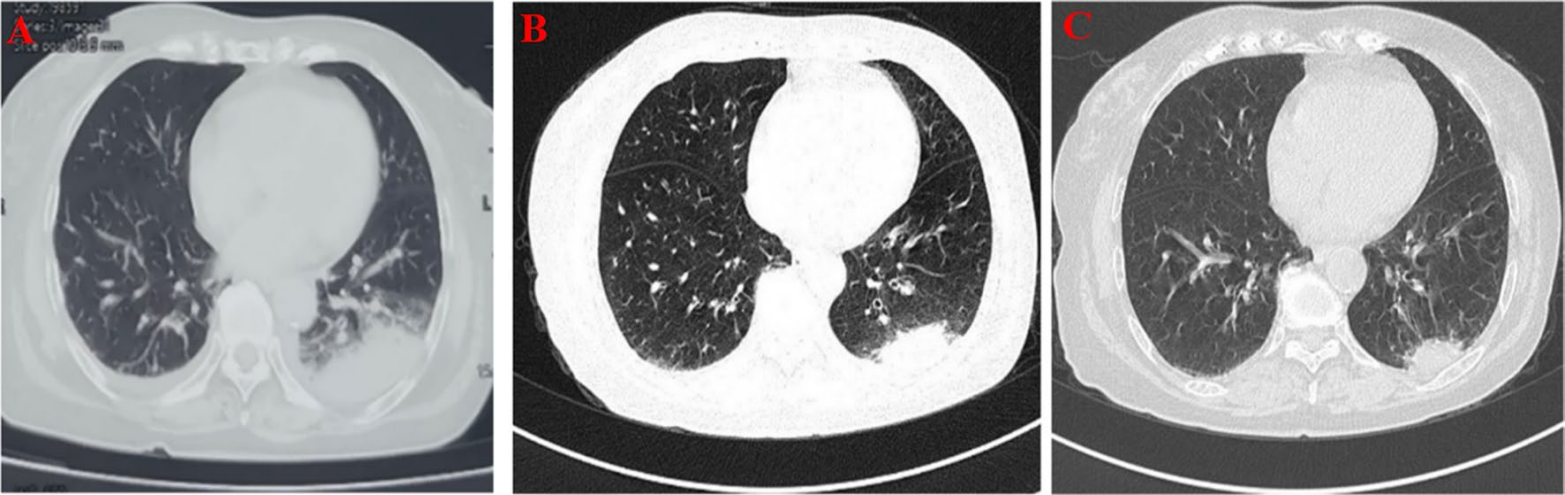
Chest computed tomography scan of the patient. The dates from left to right are 15 September 2021 (A), 23 September 2021 (B), and 29 September 2021 (C)
